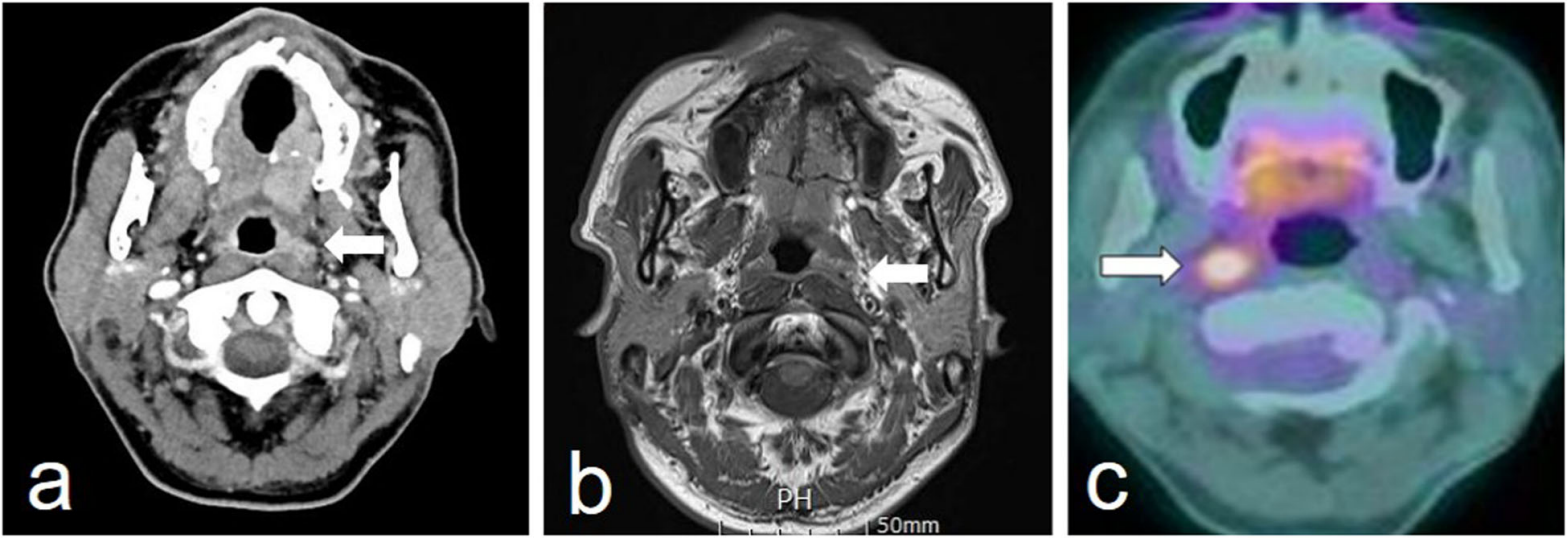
Representative false positive imaging findings of retropharyngeal lymph node. a Contrast-enhanced computed tomography shows a left false positive retropharyngeal lymph node (*arrow*). b T1-weighted gadolinium-enhanced magnetic resonance imaging shows a left false positive retropharyngeal lymph node (*arrow*). c Positron emission tomography-computed tomography shows an abnormal ^18^fluorine-fluorodeoxyglucose uptake with a standardized uptake value of 3.2, on a right retropharyngeal lymph node (*arrow*)
